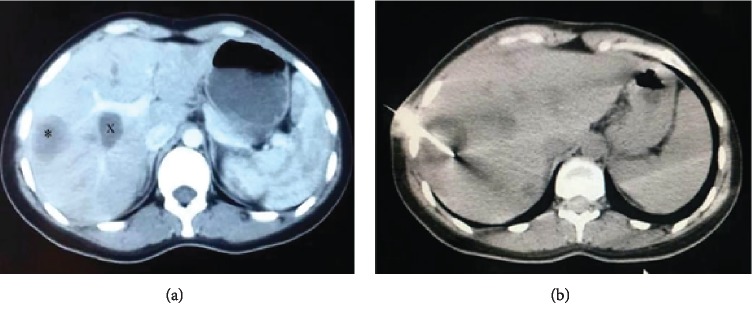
Postoperative abdominal CT scan showing (a) the remaining central hepatic cyst “x” and a remaining cavity “∗”; (b) percutaneous management of the cyst and cavity under CT guidance.
